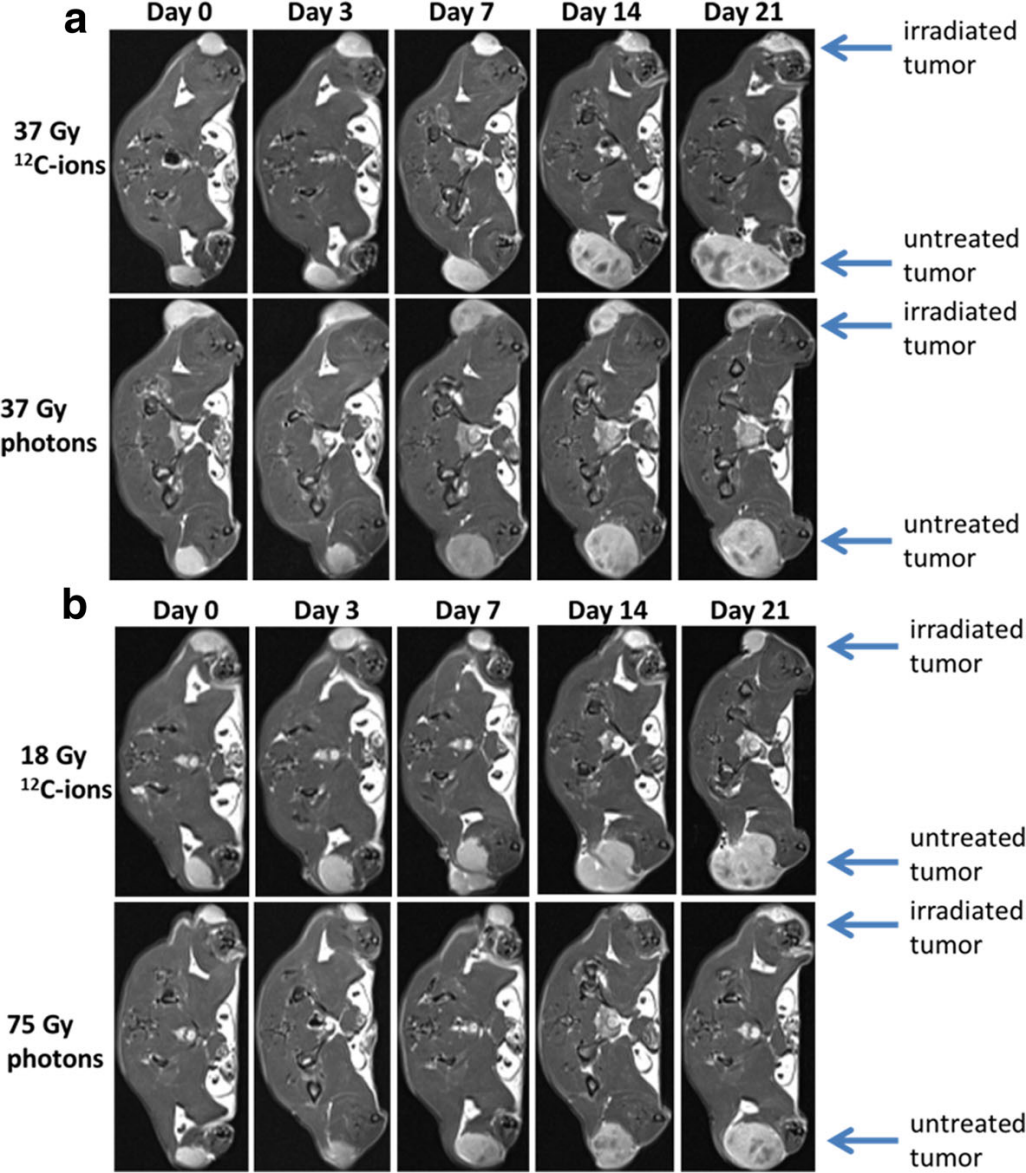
Exemplarily T1-weighted axial MR-images measured 6 min after contrast agent injection. HI-tumors were either treated (upper tumor in each image) or untreated (lower tumors). Tumors were measured before RT (Day 0) and at 4 time points after single doses. Due to the fast growth of untreated tumors, a longer follow-up was not possible. In (a) 37 Gy isodoses of photons and ^12^C-ions are compared whereas in (b) isoeffective doses with respect to local control at 300 days are shown (18 Gy ^12^C-ions vs. 37 Gy photons and 37 Gy ^12^C-ions vs. 75 Gy photons, respectively). A volume increase was seen only in untreated tumors. The light contrast showed edema, whereas dark volumes are a sign for necrotic areas
